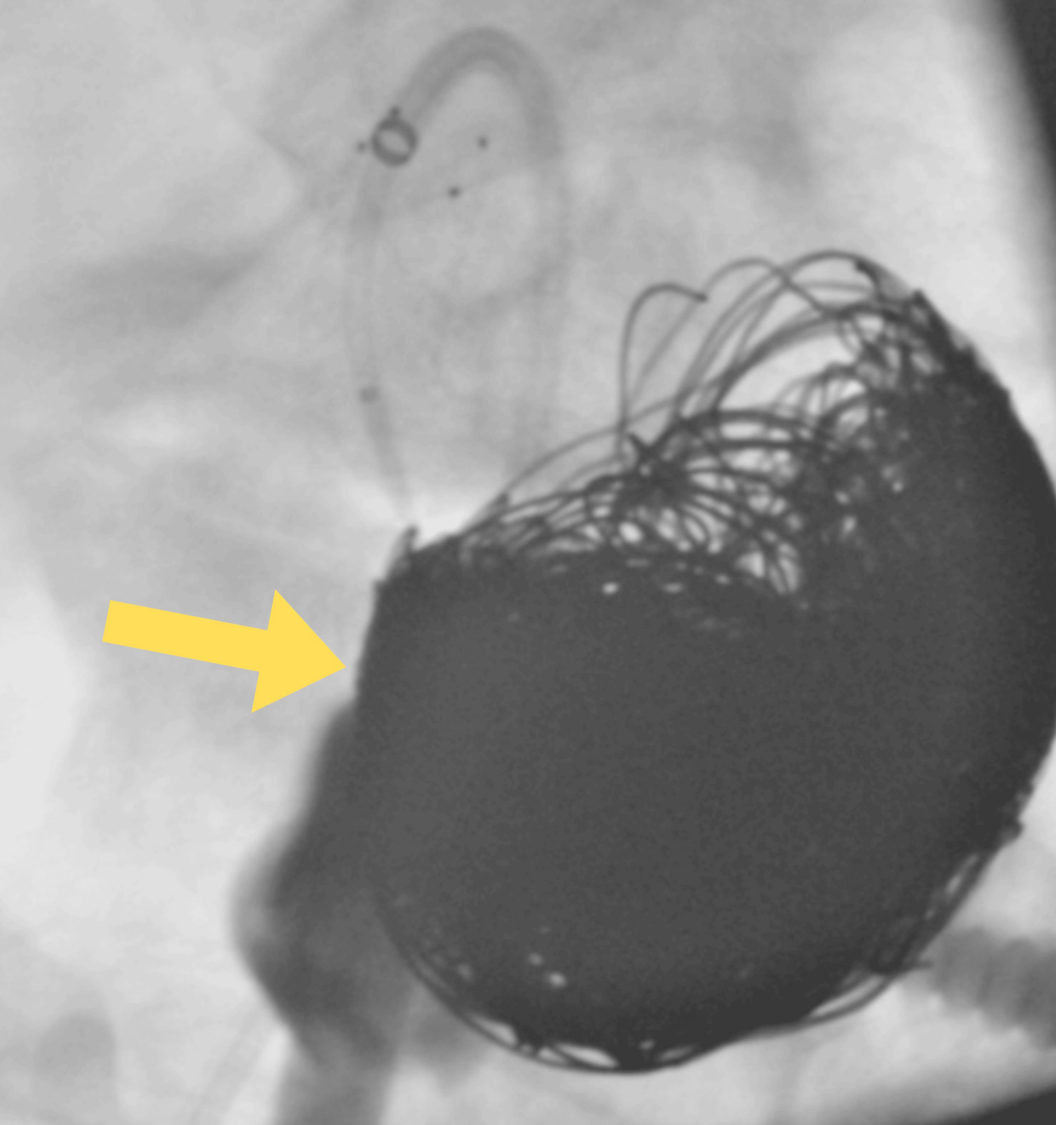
Intraoperative angio-CT, satisfactory embolization effect. The yellow arrow indicates embolization coils.
